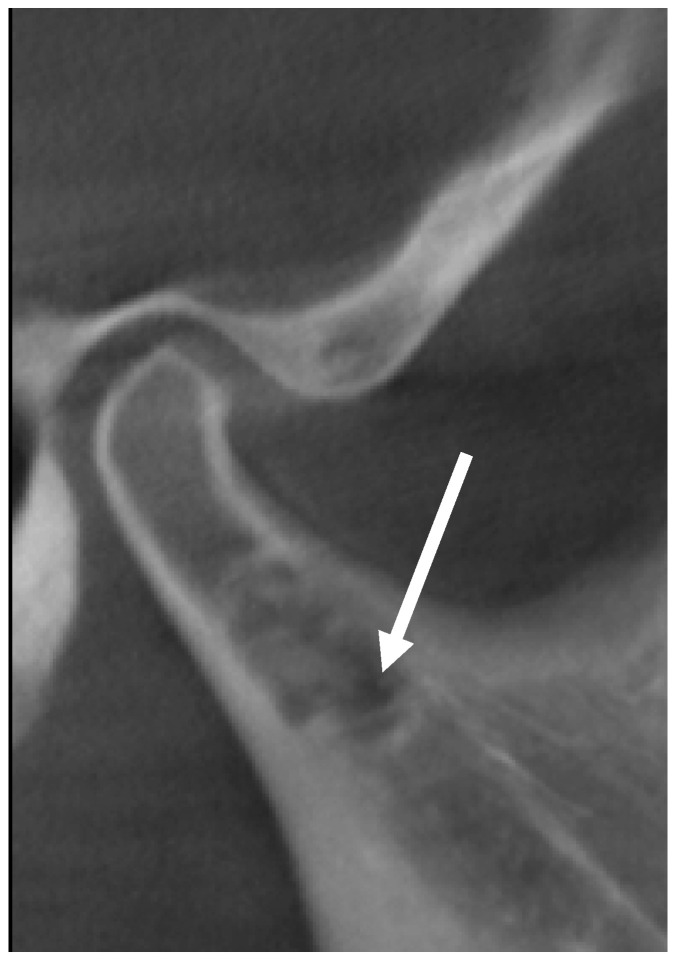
Postoperative cone-beam CT performed 6 months after surgery. Bone regeneration is evident, and only a small central area of lower bone density is not detectable (white arrow).
